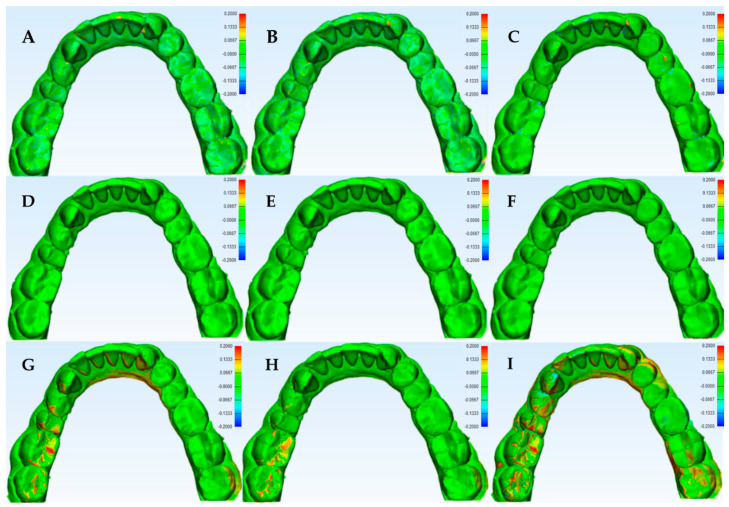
3D color histogram for precision analysis of the tested scanners in one model. (A–C) are 1st vs. 2nd, 2nd vs. 3rd, 1st vs. 3rd scans. The first row (A–C) for OS, (D–F) for CBCT and (G–I) for the IOS.
